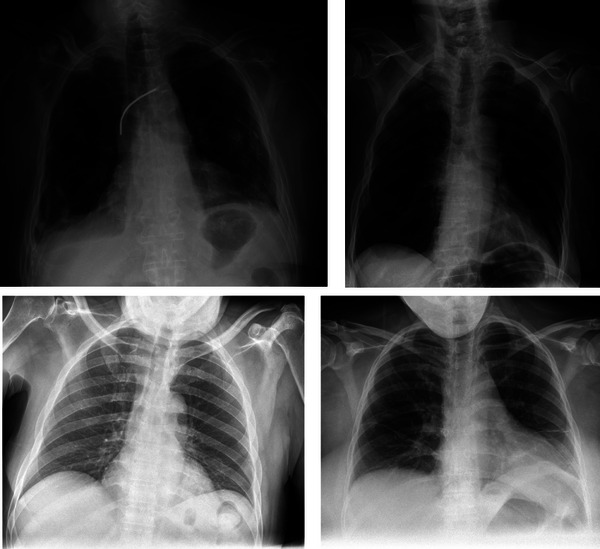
Chest radiographs demonstrating improvement in contrast following physicist intervention. Images demonstrate the difference in image appearance when using the incorrect grayscale ranger (top row), and the improvement in lung detail after updating the protocol to use the lung range selection (bottom row).
